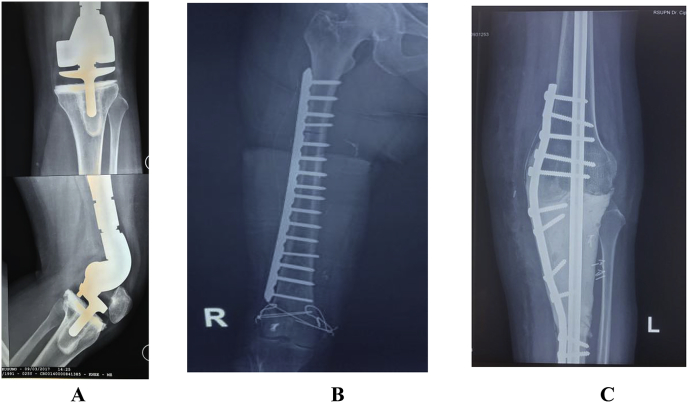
Post -operative radiographs of patients with osteosarcoma around the knee who underwent LSS: A). Megaprosthesis; B). ECI autograft and C). MAMC.
